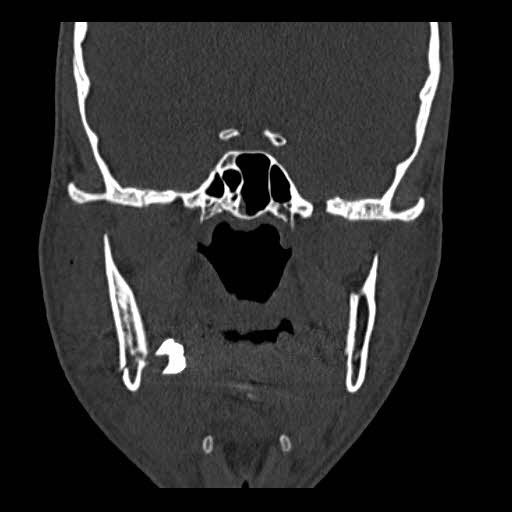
Computed tomography (CT) scan coronal view. The third molar bud was displaced to the sublingual space
